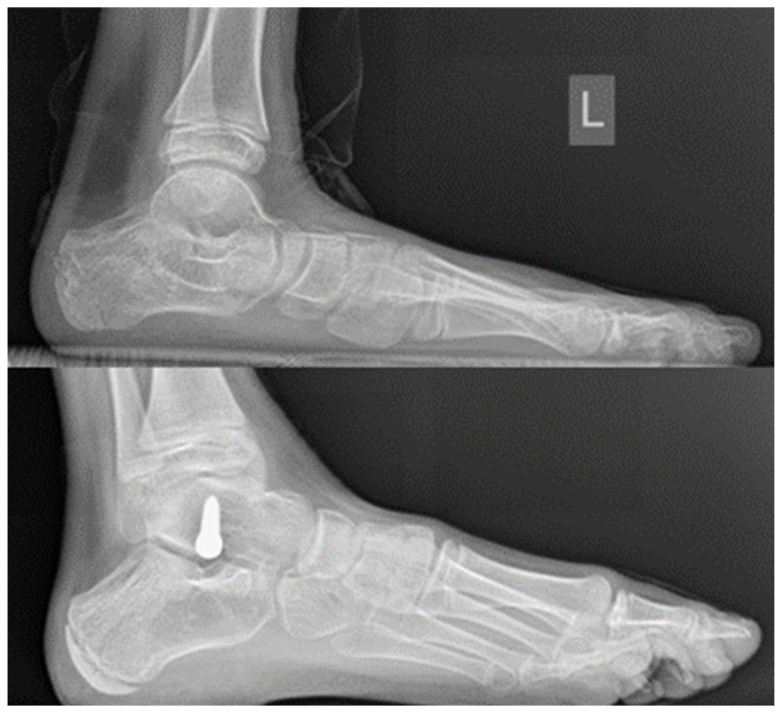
Top: pre-operative lateral radiograph demonstrating idiopathic flatfoot deformity. Bottom: intraoperative lateral radiograph confirming correct trajectory and final placement of the Spherus screw in the lateral talar tubercle.
